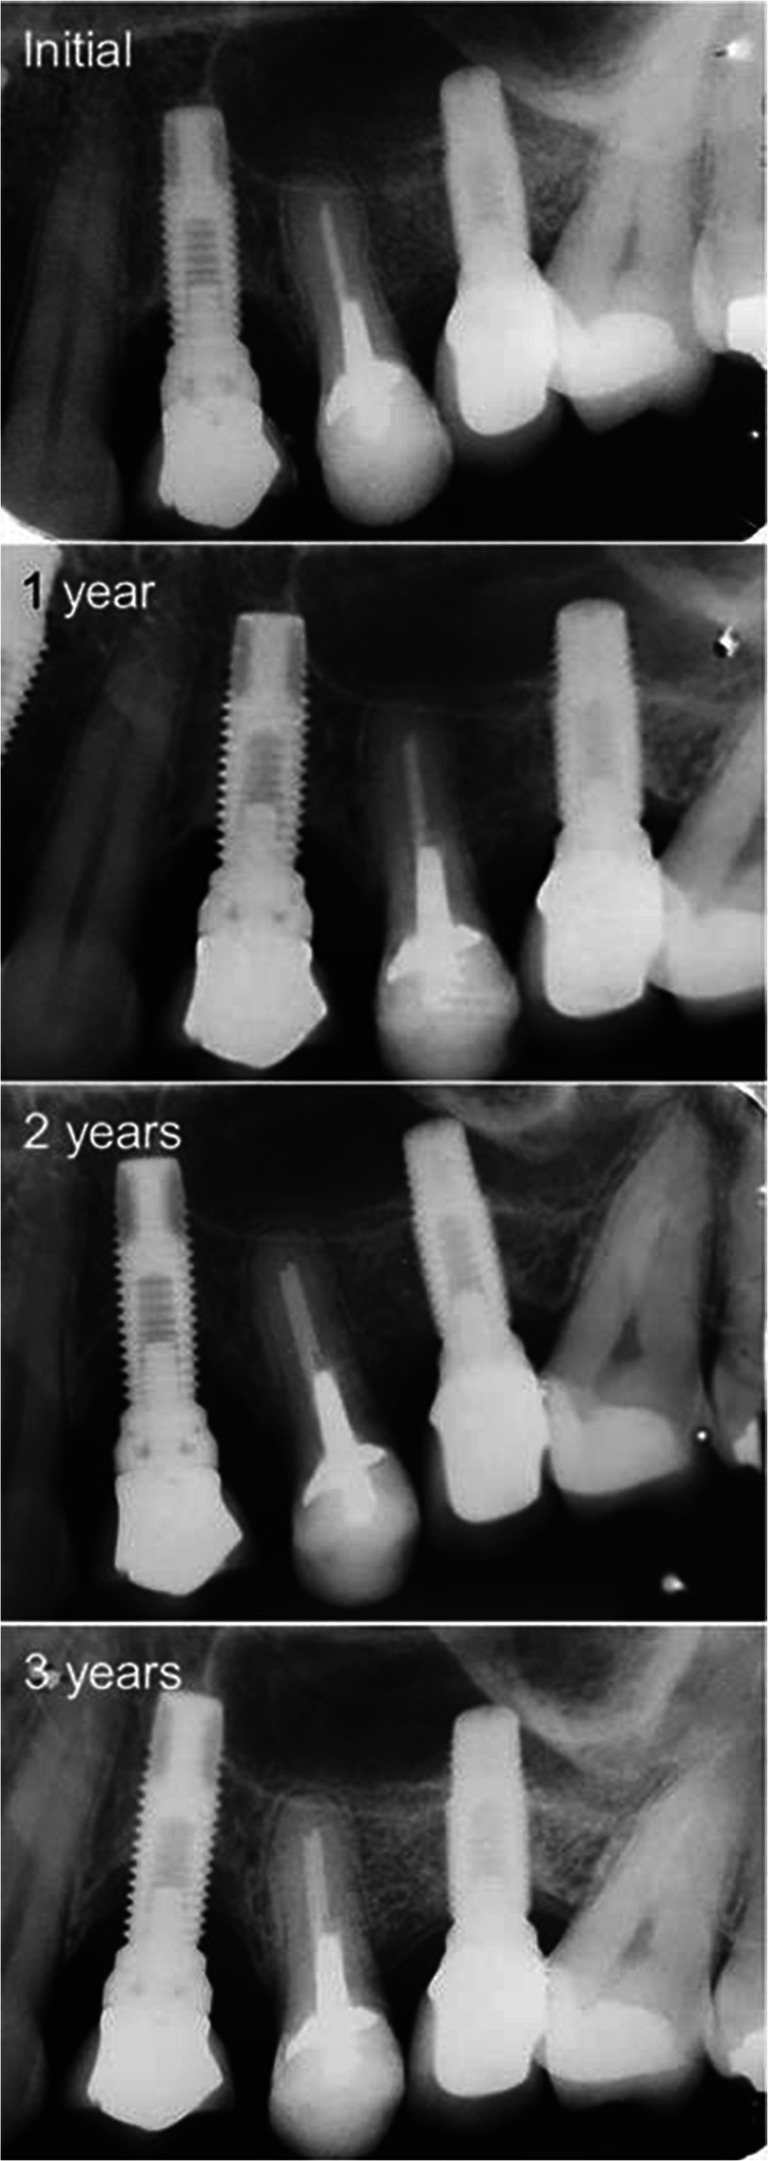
Radiographic images of two implant-supported single crowns (ISSC) at the 3-year follow-up
